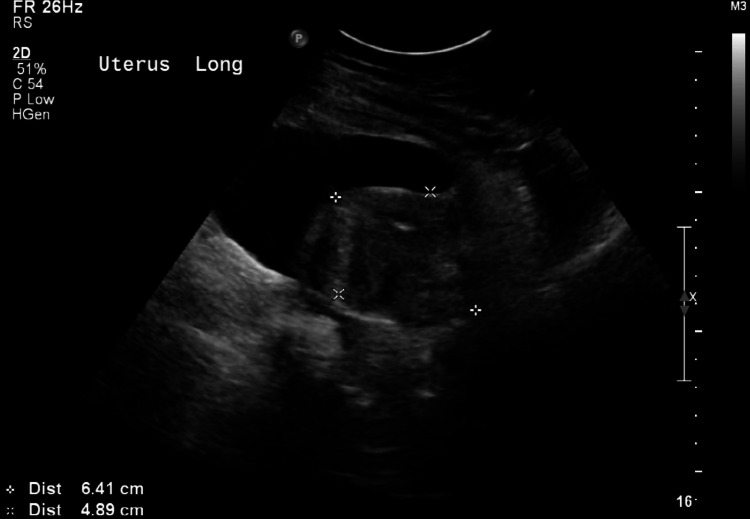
Transabdominal ultrasonography of the pelvis showing pelvic collection anterior to the uterus
